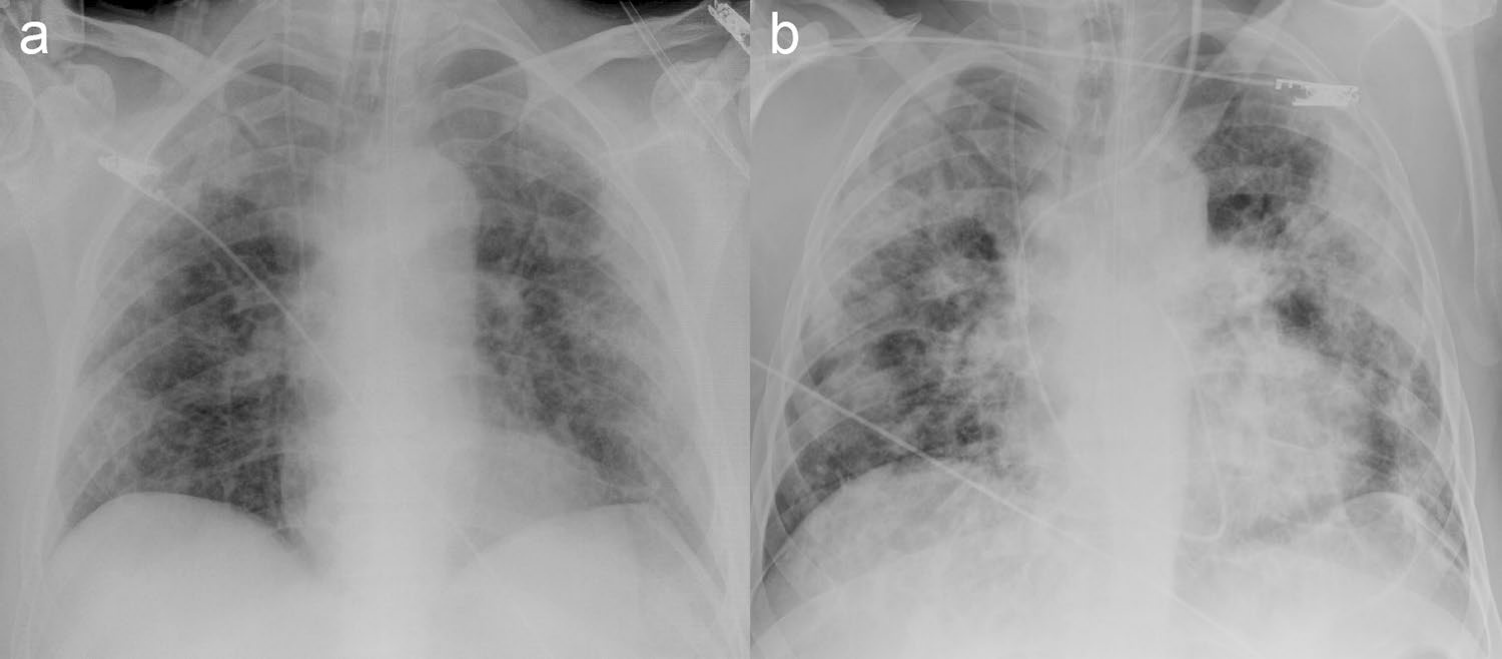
Bedside chest radiograms of a patient with a severe condition of COVID-19 pneumonia showing the temporal lung changes from the early phase (a), characterized by bilateral interstitial opacities with peripheral mid-lower lung distribution, to the peak phase (b) with bilateral and more extensive confluent alveolar opacities. Note the presence of endotracheal tube and central venous catheter in both radiographs
